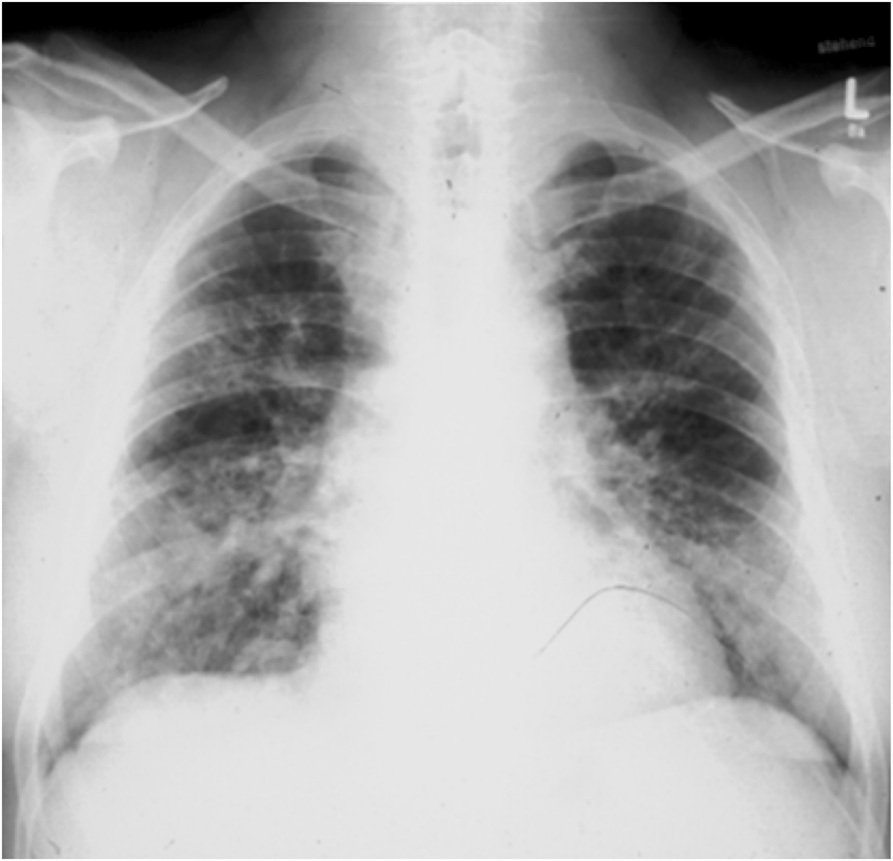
Chest x-ray of a 33 year old worker of a printing plant, suffering from subacute humidifier lung disease. For details see text. There are patchy infiltrates predominantly in the lower and middle lung fields.
